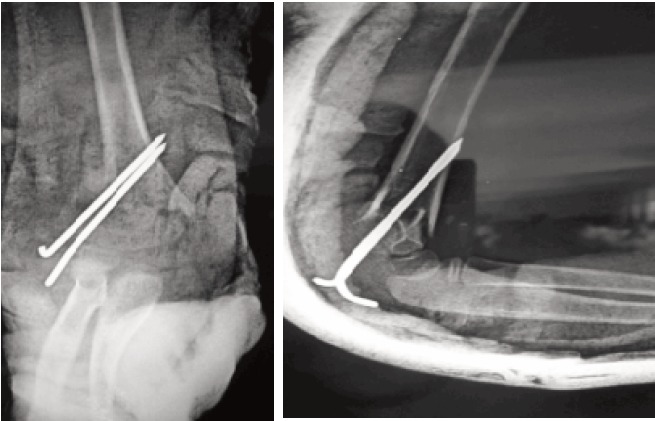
Immediate Post- operative radiograph in AP and Lateral view.
